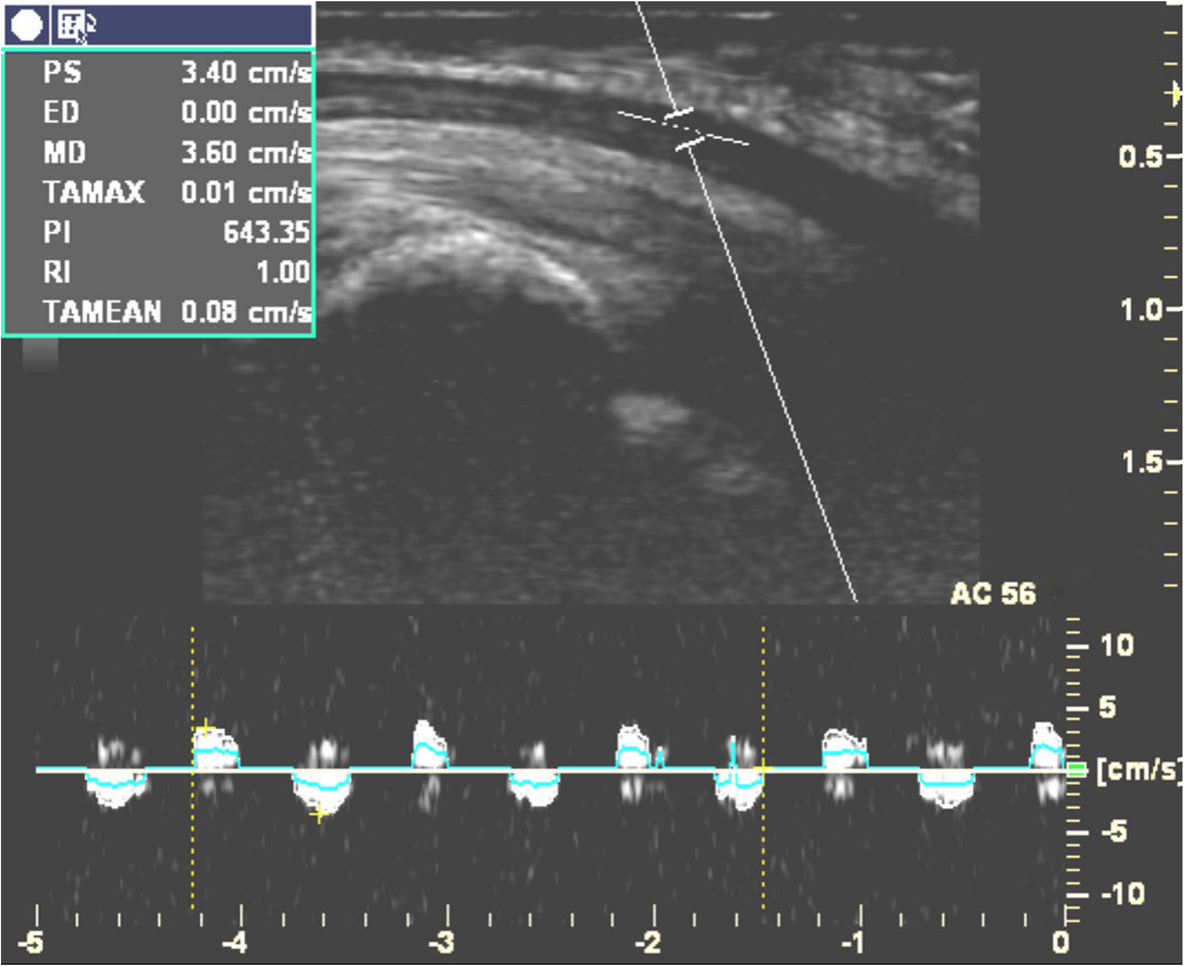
Doppler waveforms for median nerve excursion when subjects performed repetitive flexion/extension of index finger at a speed once per second
